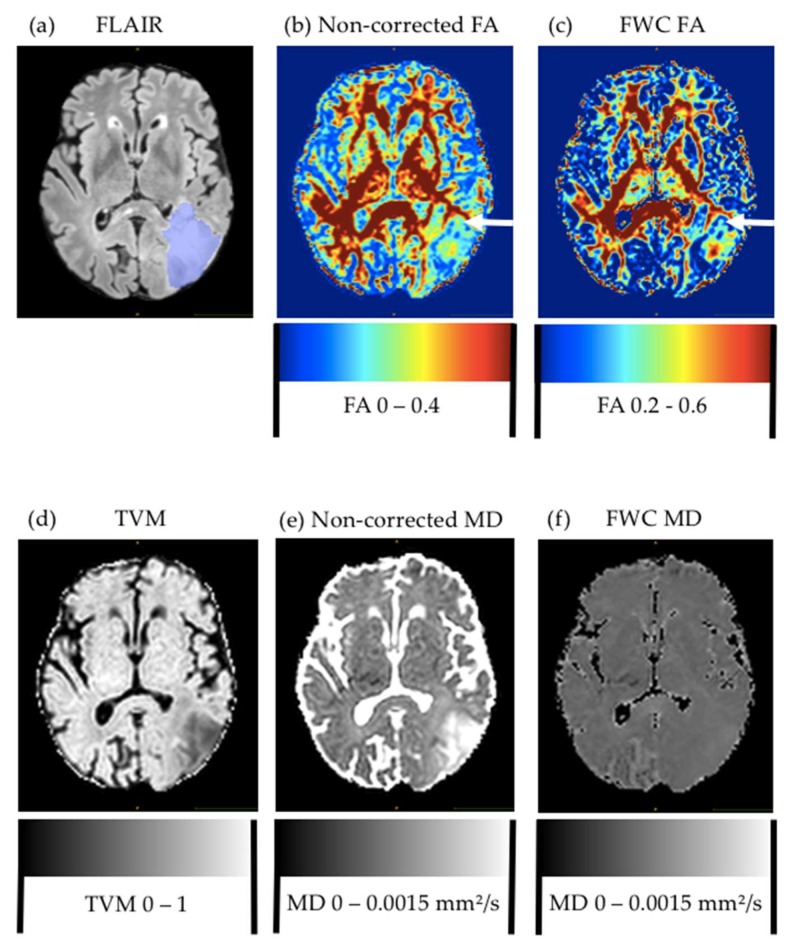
Comparison of metrics for glioblastoma patient. Preoperative FLAIR (a) with edema segmentation (overlaid in blue), and (b) noncorrected and (c) free-water-corrected fractional-anisotropy (FA) maps. Arrow in both FA maps points to area of later tumor recurrence. For improved visualization, display windows were adapted. (d) Tissue-volume map with generally lower values in peritumoral edema, but no significant differences between pure edema and edema with later recurrence. Mean-diffusivity map (e) before and (f) after free-water correction, which noticeably decreased contrast.
